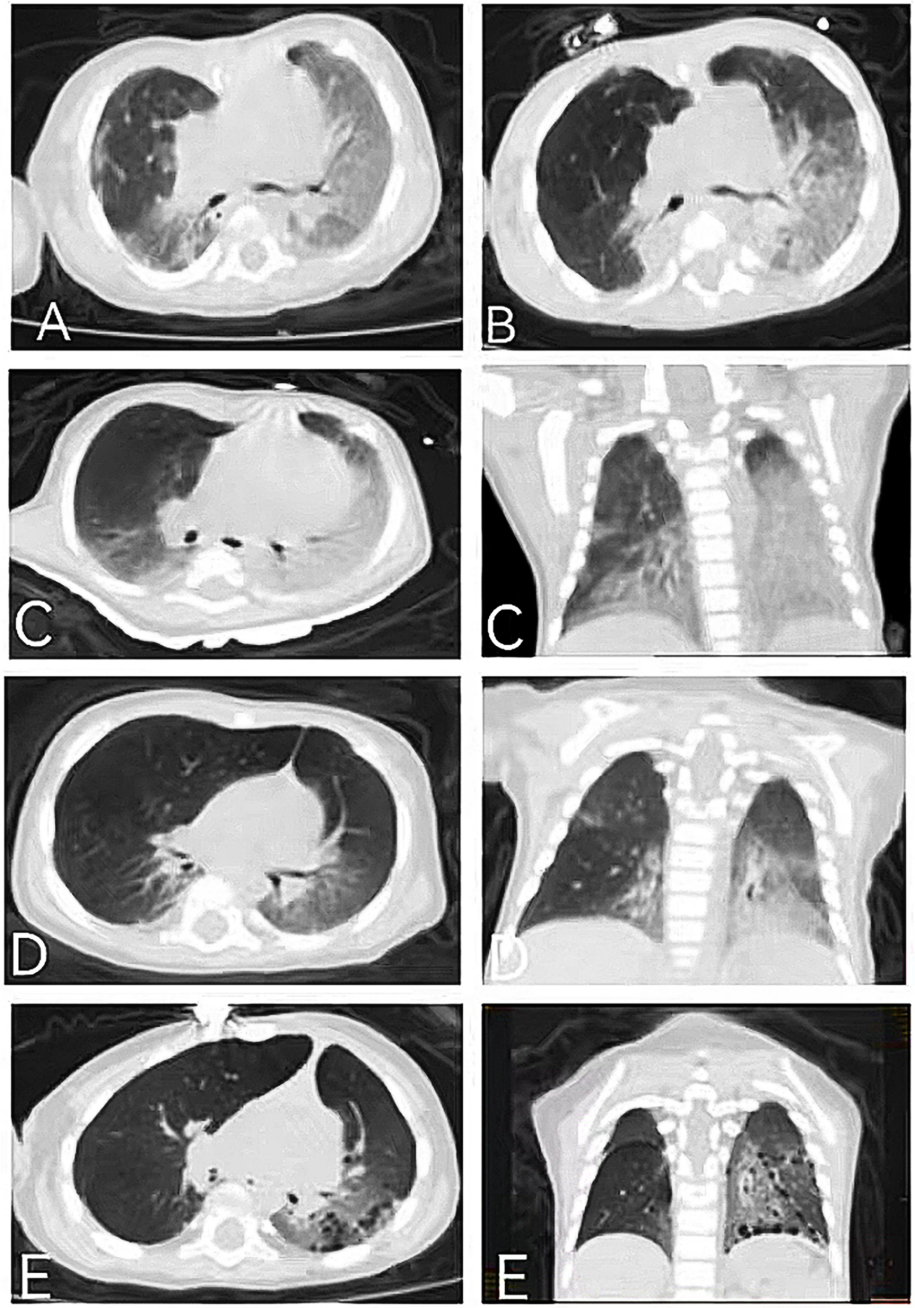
Chest CT of the elder (A,B) and younger (C–E) brothers: (A) chest CT of the elder brother showing bilateral GGOs, consolidations, and reticular opacities at the age of 3 months; (B) chest CT of the elder brother showing slightly improved GGOs but aggravated consolidations at the age of 8 months; (C) chest CT of the younger brother showing GGOs, consolidations, and mediastinal lung hernia at the age of 1 month; (D) chest CT of the younger brother showing improved GGOs and consolidations at the age of 4 months; and (E) chest CT of the younger brother showing the appearance of cysts and improved GGOs and consolidations at the age of 14 months.
